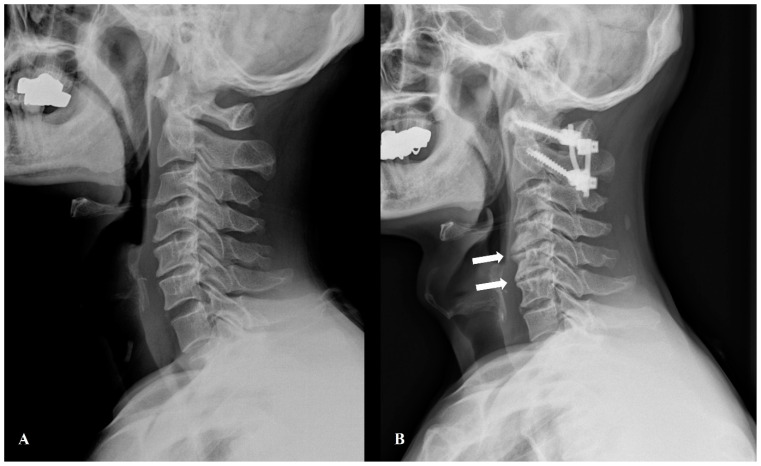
Preoperative plain radiograph (A) and radiologic subaxial pathology (white arrows) on postoperative plain radiograph (B).
